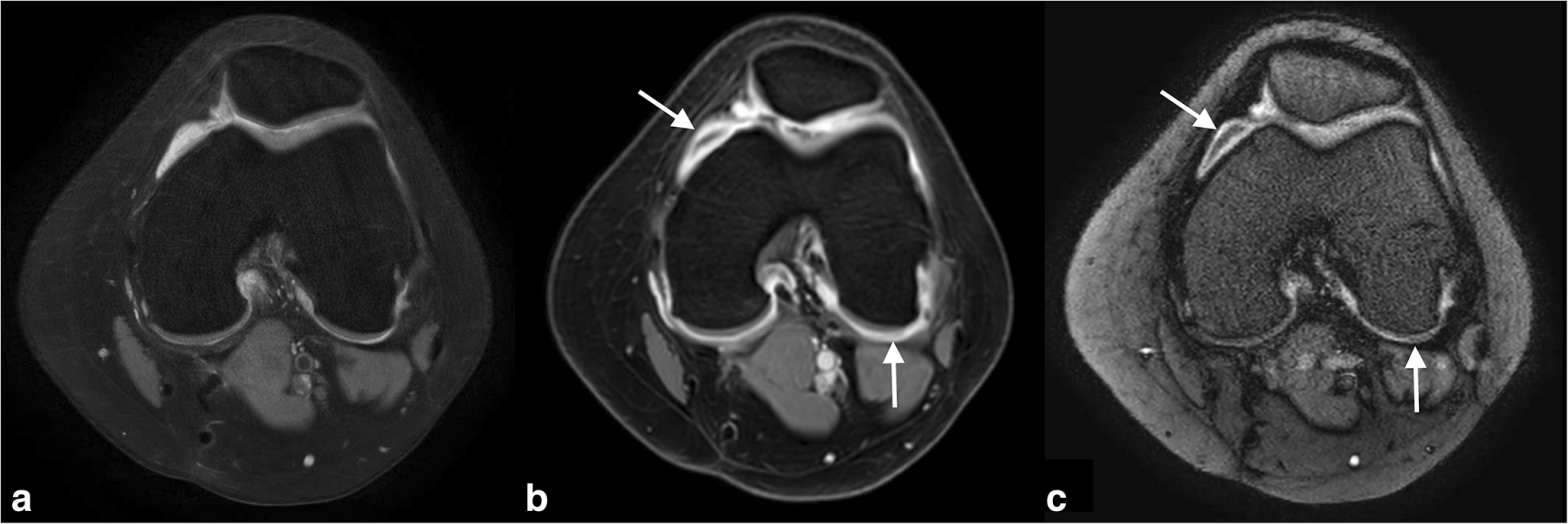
A 14-year-old boy with oligo-articular JIA and knee arthritis. MRI of the knee with (a) an axial T2-weighted fat-saturated image, (b) an axial T1-weigted fat-saturated post-Gd image showing an enhancing thickened synovial membrane retro-patellar (arrow) and posterior of the condyles (arrow) and (c) an axial double inversion recovery (DIR)–weighted Gd-free image showing a similar picture (arrows)
